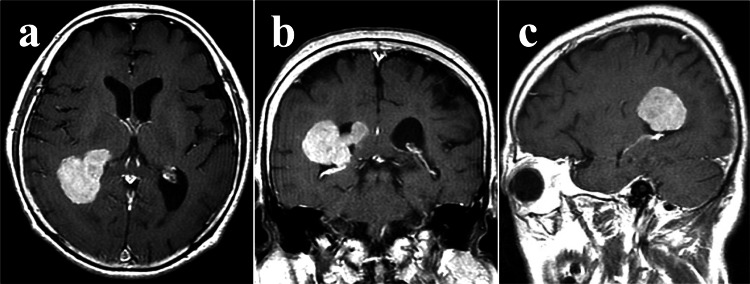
Preoperative gadolinium-enhanced MRI showing a meningioma in the right posterior horn of the lateral ventricle. (a) Axial, (b) Coronal, and (c) Sagittal views MRI; magnetic resonance imaging
